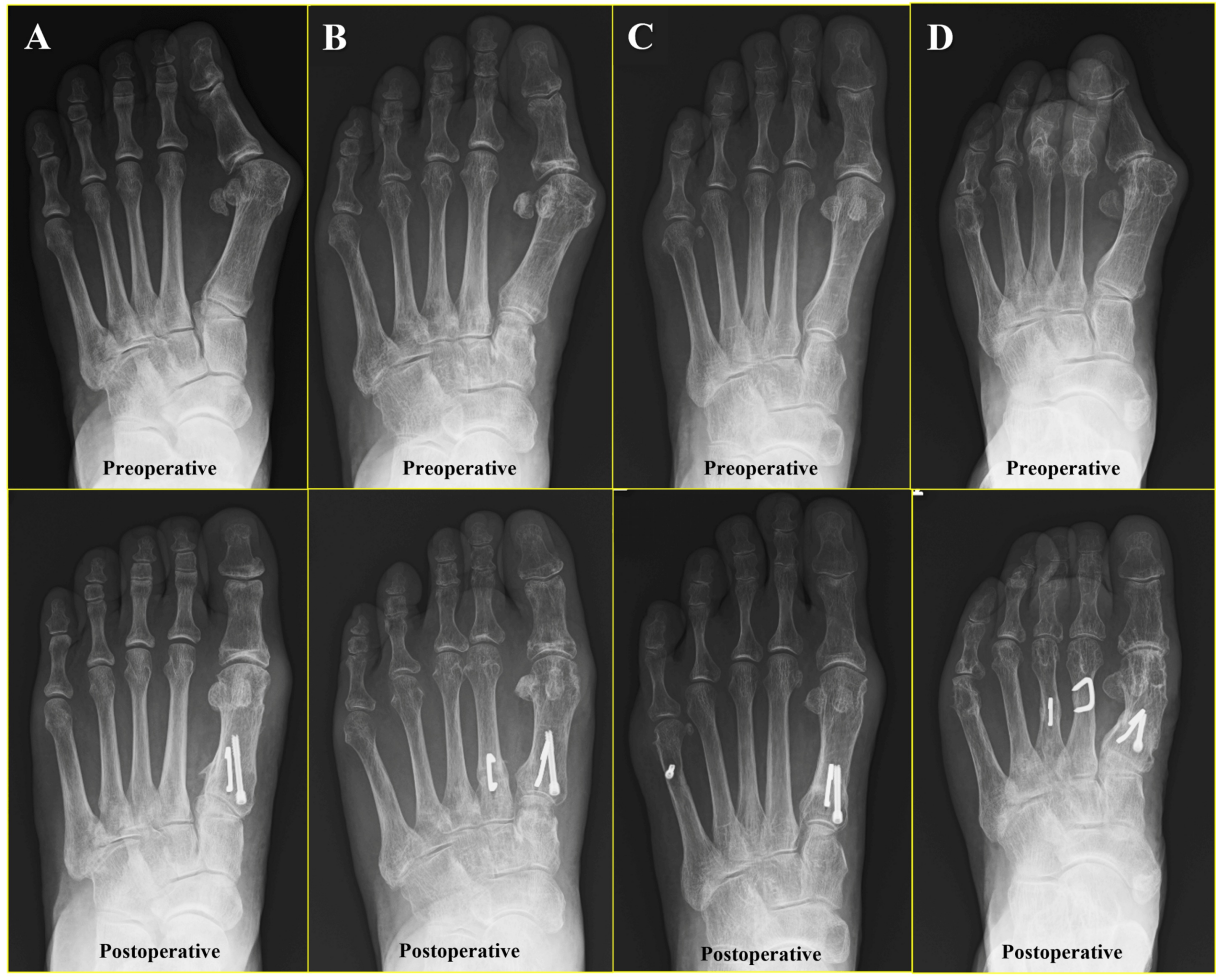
Representative plain radiographs of each procedure. (A) Isolated PCWO. (B) PCWO with shortening of the second metatarsal. (C) PCWO with oblique diaphyseal osteotomy. (D) PCWO with multiple surgeries for lesser toes.
